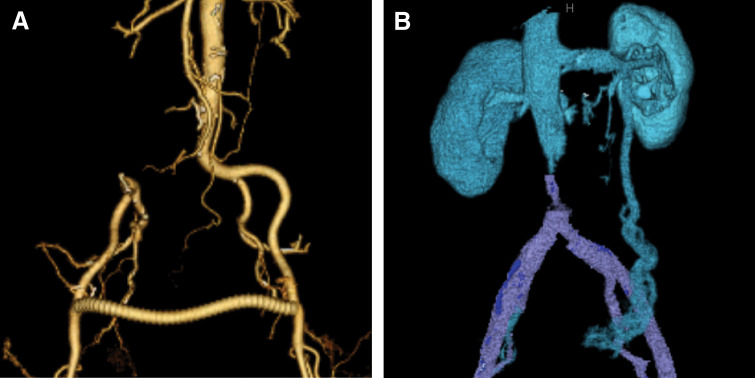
Postoperative CTA showing reconstructed vascular anatomy. (A) Arterial reconstruction image demonstrating preserved blood flow to the right internal and external iliac arteries via a femoral-to-femoral bypass graft. (B) Venous reconstruction image showing inferior vena cava stenosis, with the left ovarian vein serving as a collateral pathway.
